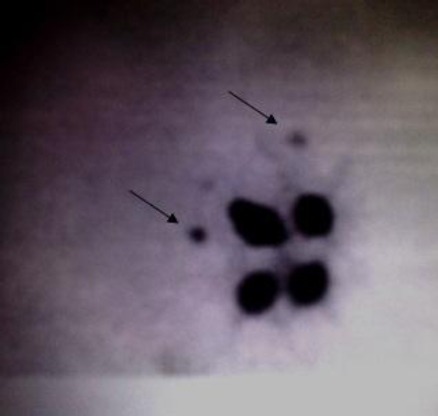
Dual method of scintigraphic SLN detection using 99mTc-SENTI-SCINT and blue dye injection - AP position 2 h post injection*.
